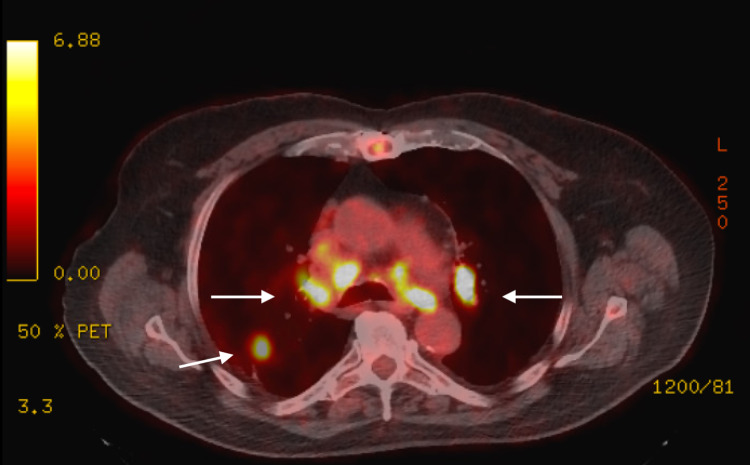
PET scan Intense FDG uptake among right upper lobe nodule and bilateral mediastinal lymph nodes (white arrows) PET, positron emission tomography; FDG, fluorodeoxyglucose
